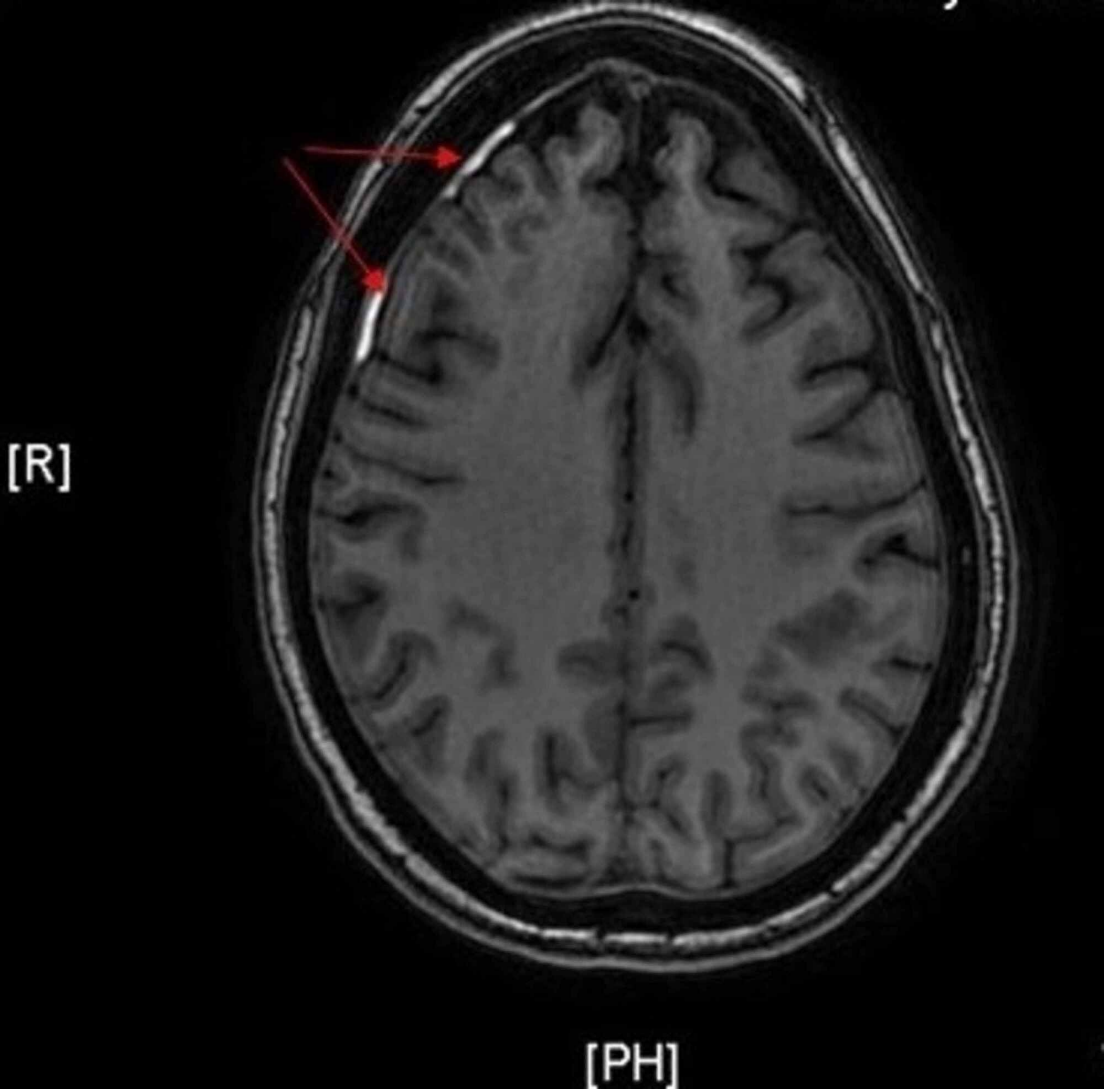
MRI of the brain with T1 hyperintensity (red arrows) consistent with a thin subdural hematoma, 3 mm in maximal thickness
